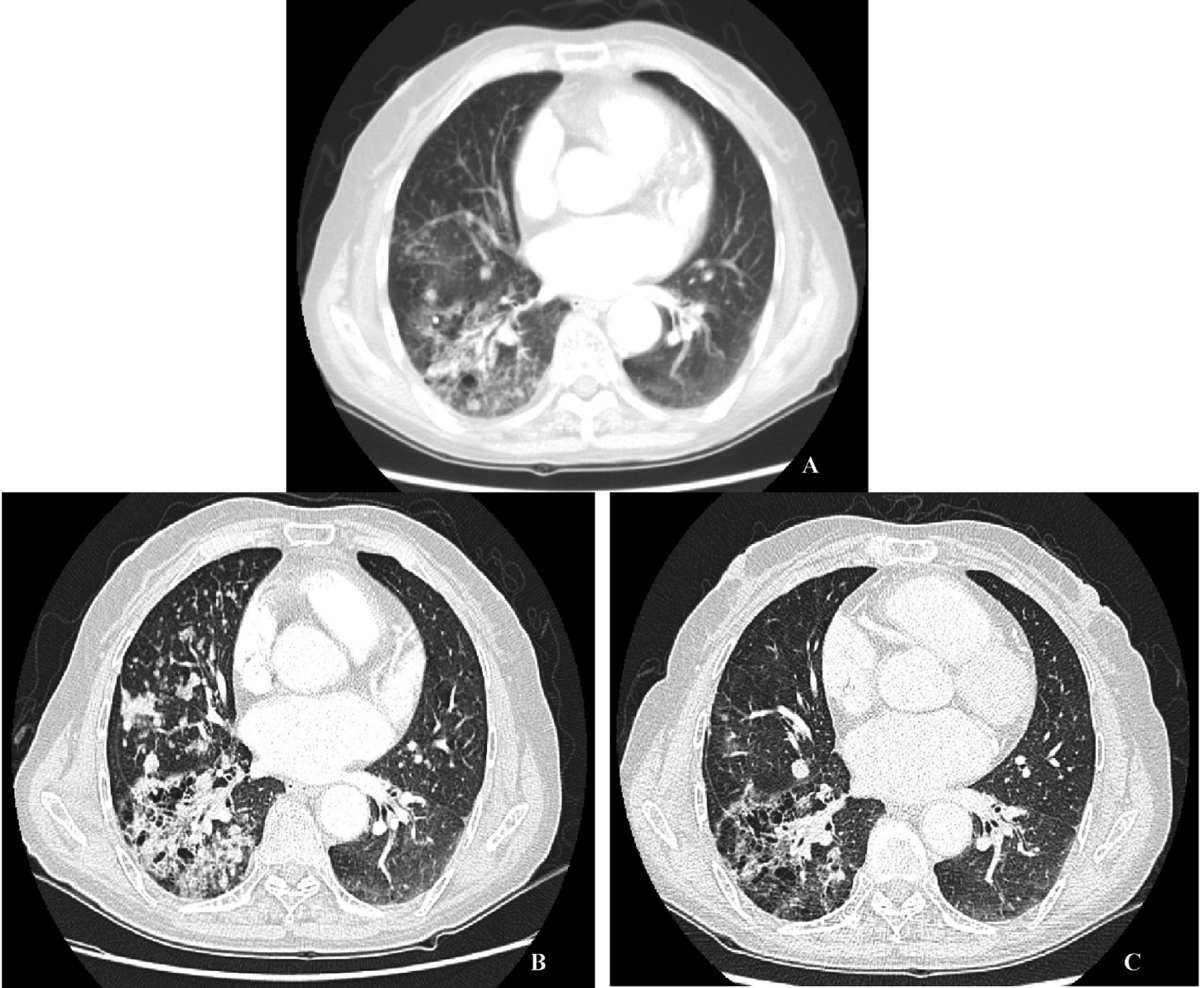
A: Chest CT scan after treatment with 2 courses of pemetrexed, more aggravated consolidative mass in RLL and more enlarged metastatic nodules in RML and RLL. B: Chest CT scan after treatment with 4 courses of gemcitabine/vinorelbine, more aggravated consolidative mass in RLL and more enlarged metastatic nodules in both lungs. C: Chest CT scan 2 months after re-treatment with 250 mg gefitinib, slightly decreased consolidative mass in RLL and markedly decreased metastatic nodules in both lungs.
